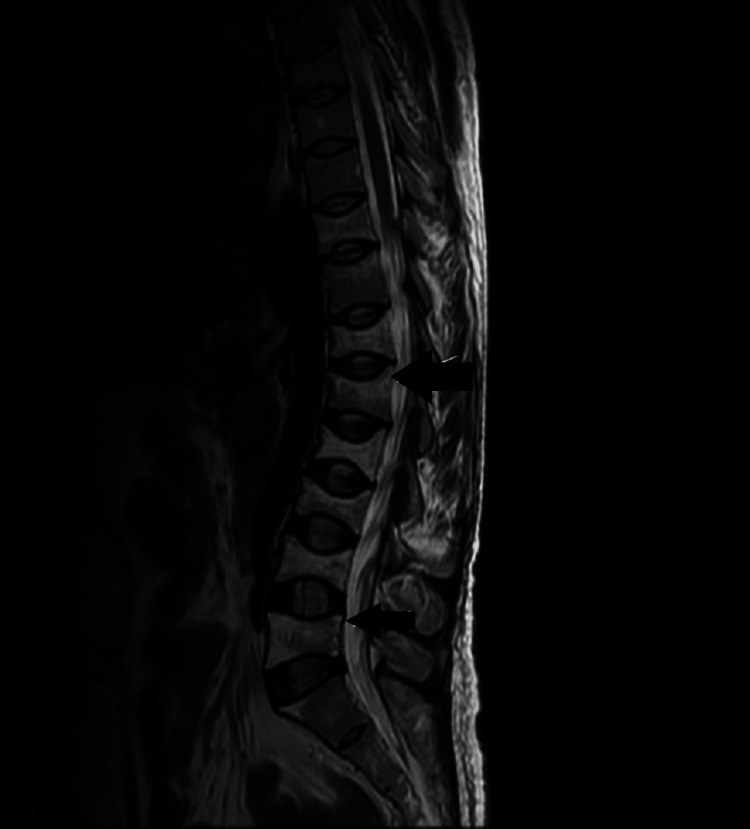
MRI of the spine in sagittal view The arrows indicate the biconcave appearance of T10-T12 vertebrae and all five lumbar vertebrae, fatty marrow replacement in all the lumbar vertebrae and T10-T12 vertebrae, exaggerated kyphosis at L1 and L2, mild scoliosis at the thoracolumbar spine, and increased convexity to the right
